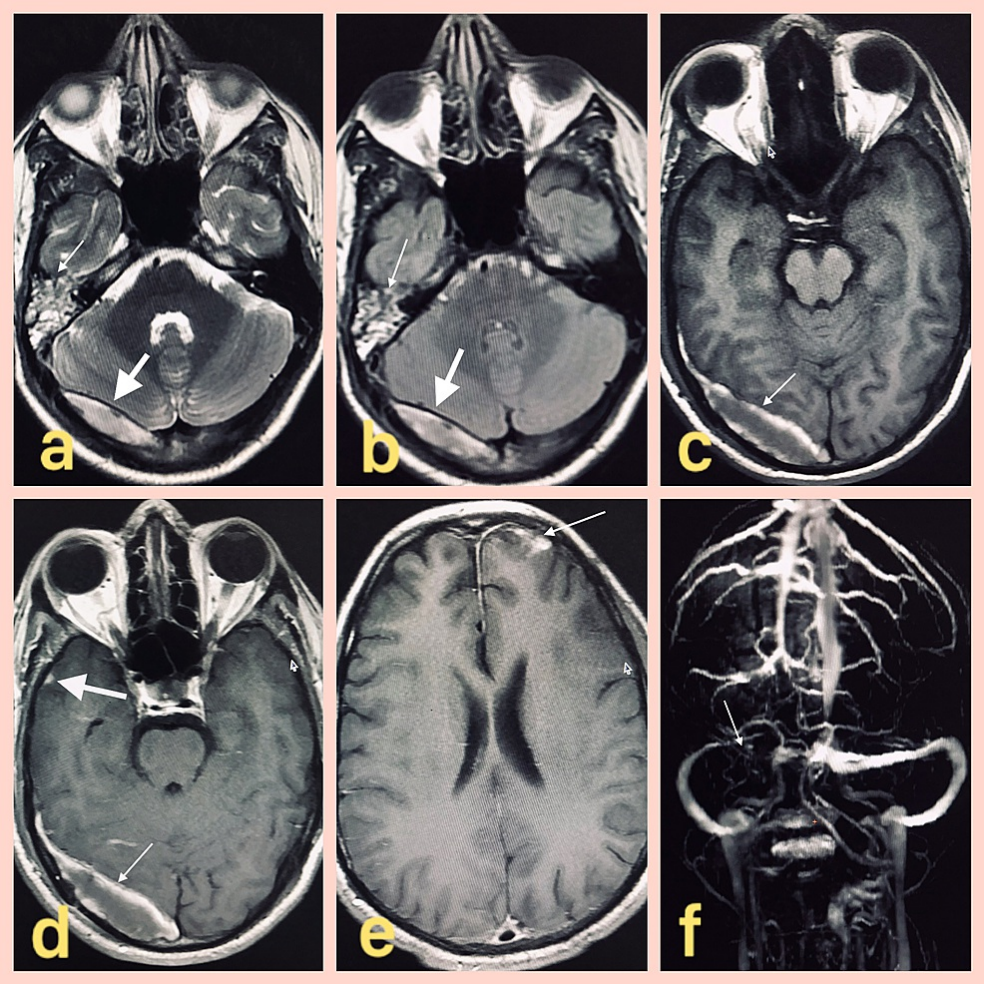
Axial T2WI, T2-FLAIR, T1 non-contrast, T1WI post-contrast, 3D TOF-MRV images of the brain. (a) Axial T2WI of the brain showing fluid intensity in the right mastoid air cells (denoted by thin white arrow) and T2 hyperintense distended right transverse sinus (denoted by thick white arrow). (b) Axial T2 FLAIR of the brain showing similar signal characteristics involving the right mastoid air cells (denoted by thin white arrow) and right transverse sinus (denoted by thick white arrow). (c) Axial T1 non-contrast image showing distended right transverse sinus (denoted by white arrow). (d) Axial T1WI post-contrast image showing a non-enhancing filling defect in the right transverse sinus (denoted by thin white arrow) and a focus of abnormal parenchymal enhancement in the right temporal lobe (denoted by thick white arrow). (e) Axial T1WI post-contrast images showing a nodular focus of leptomeningeal enhancement in the left frontal lobe (denoted by thin white arrow). (f) 3D TOF-MRV image showing the non-visualization of the right transverse sinus and portion of the right sigmoid sinus (denoted by white arrow). T2WI: T2-weighted image; T1WI: T1-weighted image; T2-FLAIR: T2-weighted-fluid-attenuated inversion recovery; TOF-MRV: time of flight-magnetic resonance venography
